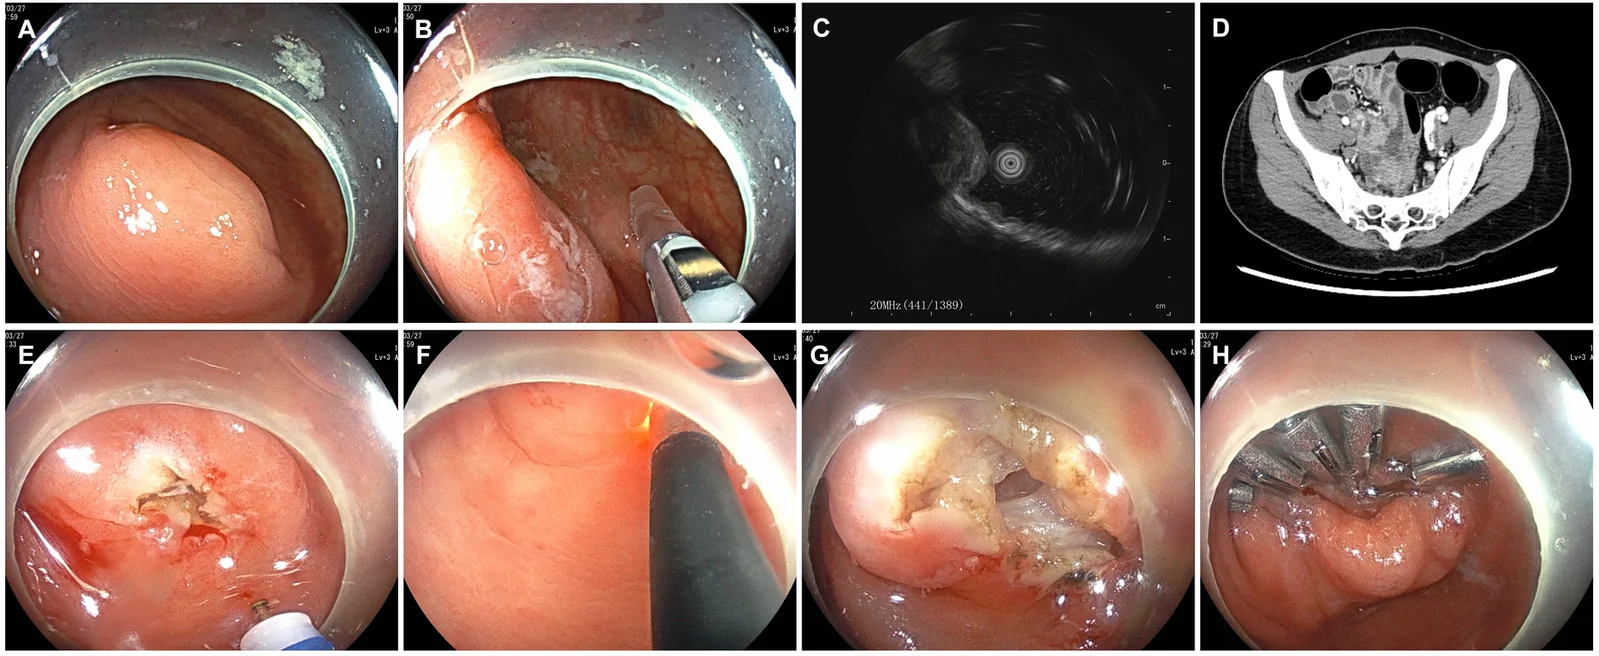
Imaging features and endoscopic treatment of periappendiceal root abscess. (A) Colonoscopy reveals a submucosal protuberance beside the appendiceal opening. (B and C) Endoscopic ultrasonography visualizes an extramural lesion originating outside the fourth intestinal wall layer with heterogeneous internal echoes and localized hypoechoic areas; fluid discharge can be seen after compression on the lesion. (D) Contrast-enhanced CT displays a well-demarcated hypodense lesion at the appendiceal base. (E) An IT knife is applied to make a central cut on the protruding lesion when the original appendiceal ostium cannot be recognized. (F) Purulent fluid drains out, subsequent saline lavage is conducted, and the cavity condition is observed via microendoscopy. (G) A 3-mm microperforation was detected intraoperatively on the intact bowel wall near the appendiceal opening. (H) An inflammatory mucosal break is found during the operation, and this break as well as the surgical incision are closed with titanium clips.
